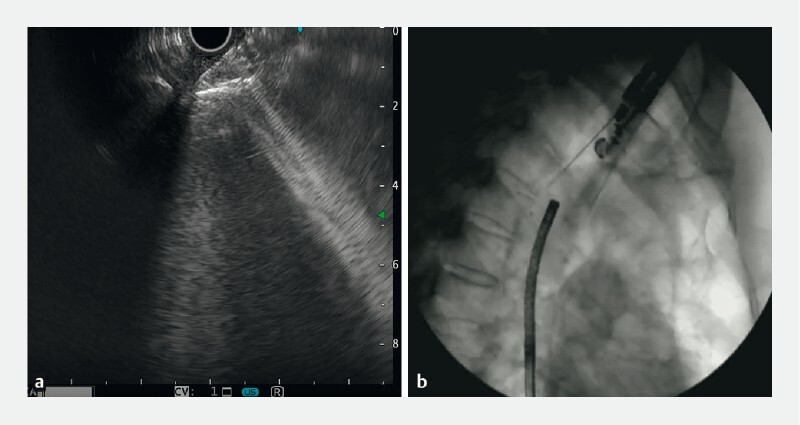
Endoscopic ultrasound (EUS)-guided fine-needle aspiration was performed to reconnect the esophagus and stomach.
